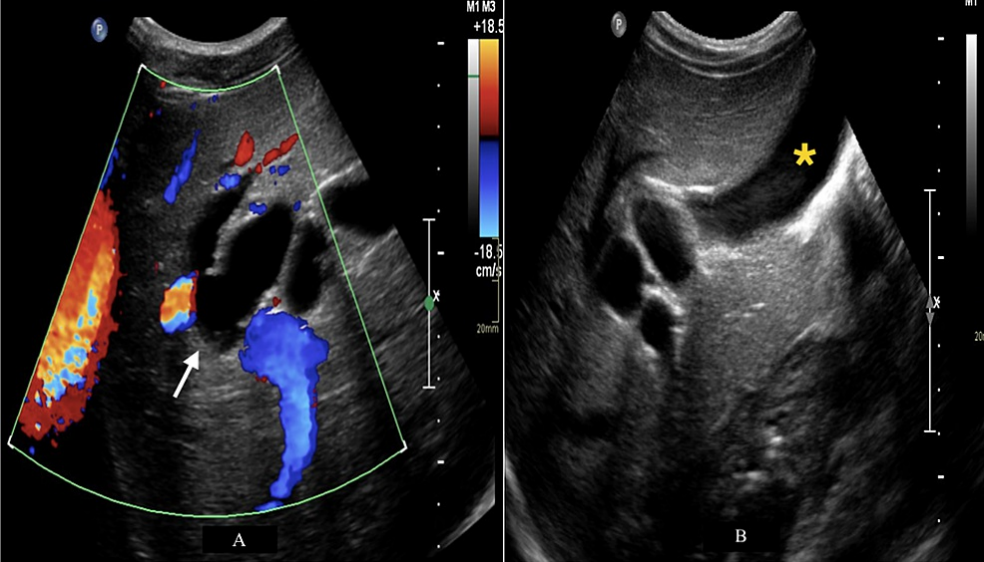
Axial ultrasound images of the liver and biliary tree Axial ultrasound images of the liver and biliary tree with doppler (A) and gray-scale (B) using a 5 MHz curvilinear probe showing cystic dilatation of intrahepatic bile ducts (A) (white arrow) with a normal gallbladder (B) (yellow asterisk). The common bile duct could not be visualized with certainty. The pancreatic duct (not shown) was of normal caliber.
